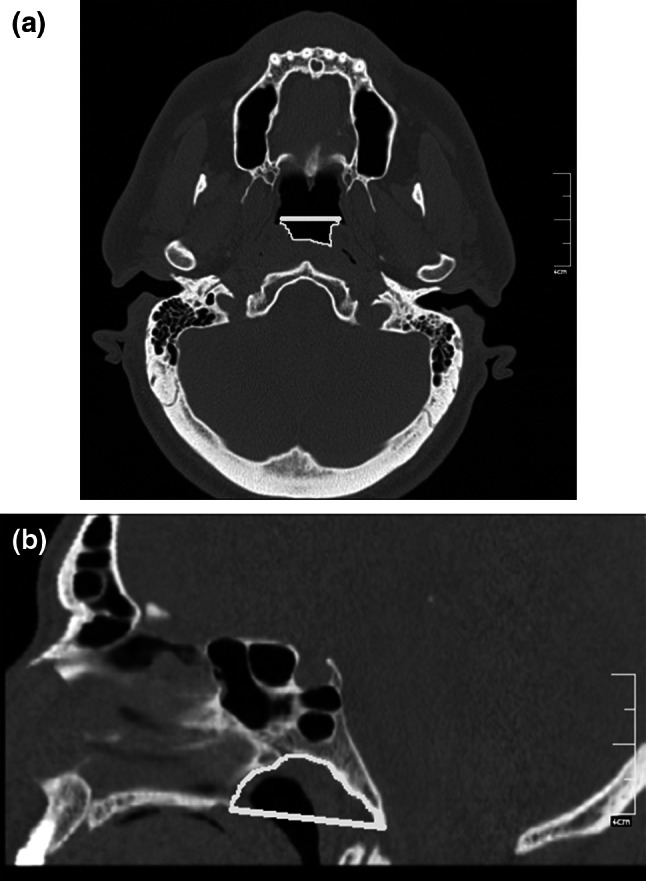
Reformatted computed tomography images. a Oblique axial image showing nasopharynx axial area (*Npax*) and the distance between the midpoints of the pharyngeal openings (*Mppod*). b Midsagittal image showing nasopharynx sagittal area (*Npsag*)
